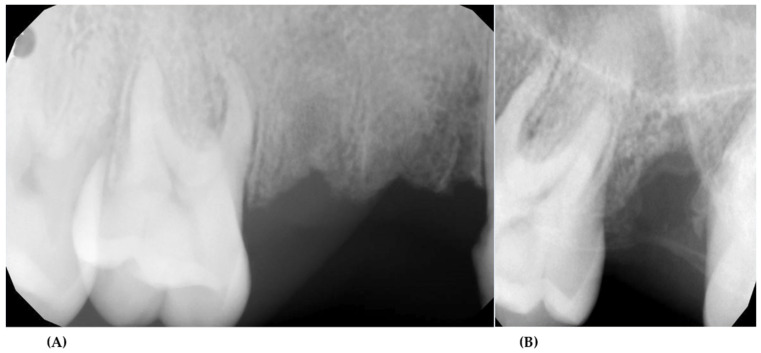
Dental X-rays—7th months after extraction: side after 14 and 15 teeth extraction—normal bone healing (A); side after 27 extraction—abnormal bone healing (B).
